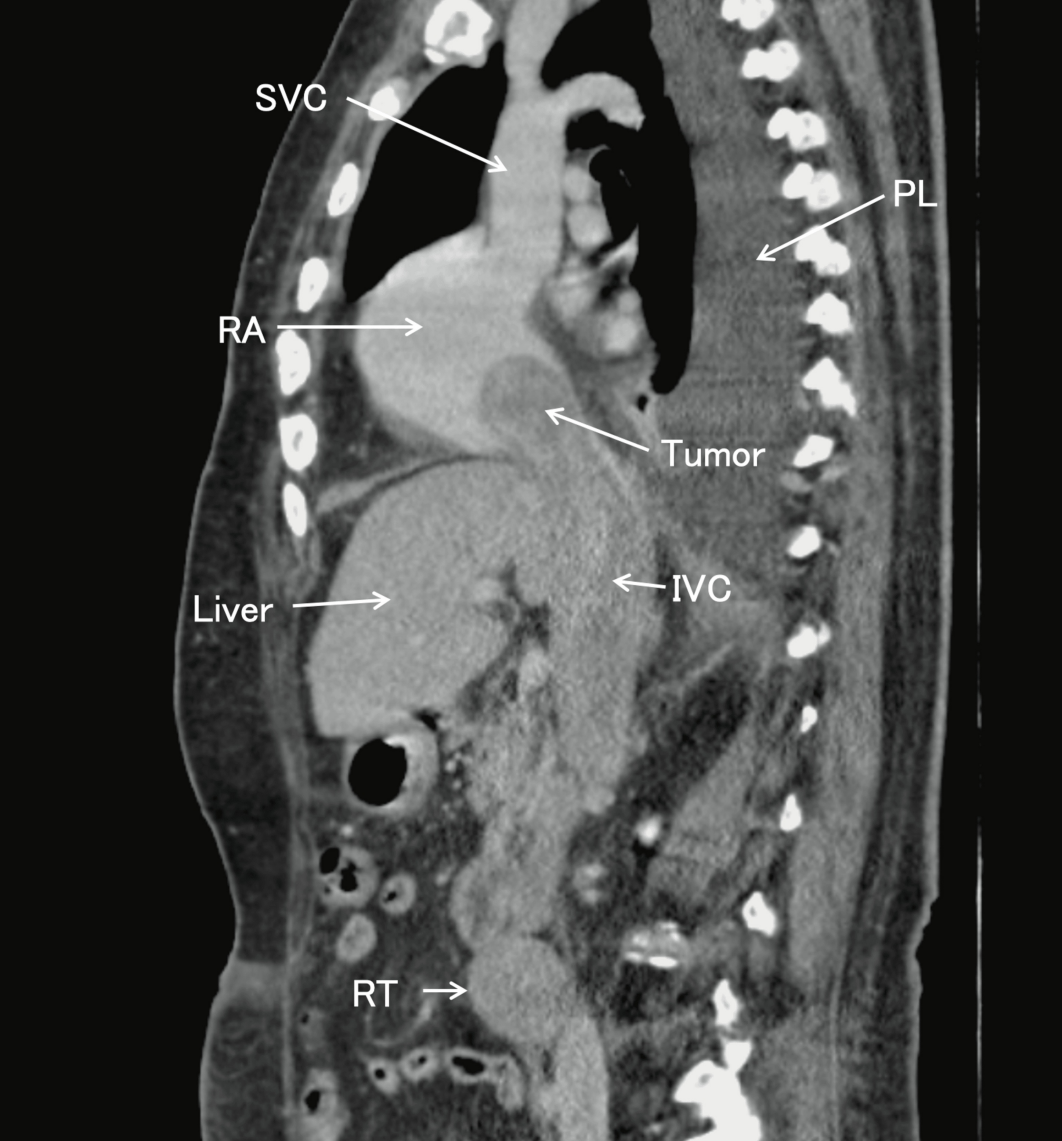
The sagittal plane of contrast-enhanced delayed phase chest and abdominal computed tomography The site of tumor recurrence is found in the retroperitoneum, invading the inferior vena cava and extending into the cardiac cavity. The inferior vena cava is filled with tumors. Pleural effusion is observed in the right thoracic cavity. SVC, superior vena cava; RA, right atrium; PL, pleural effusion; IVC, inferior vena cava; RT, site of tumor recurrence
